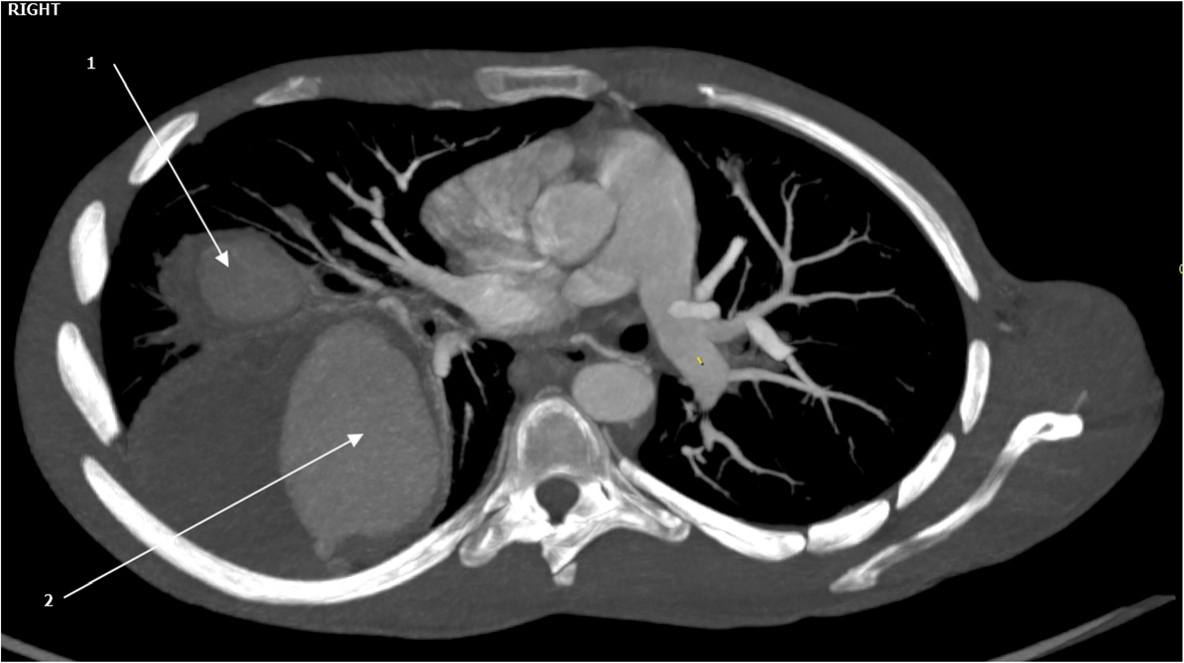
Computed tomography chest scan (axial and coronal views) showing multiple bilateral pulmonary artery pseudoaneurysms (*arrows*) with surrounding hematoma
